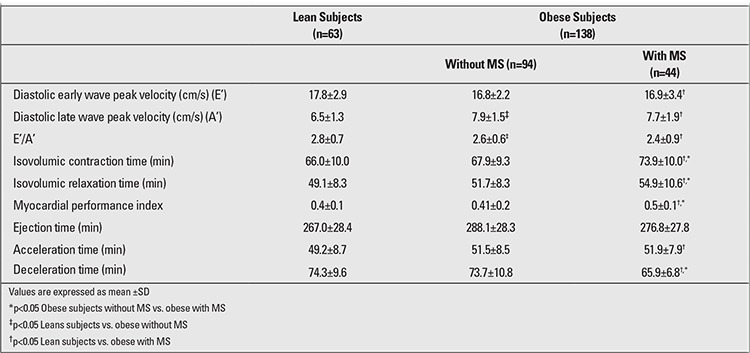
Tissue Doppler echocardiography measurements in the lean subjects and in the non- metabolic syndrome (MS) and MS obese subjects
